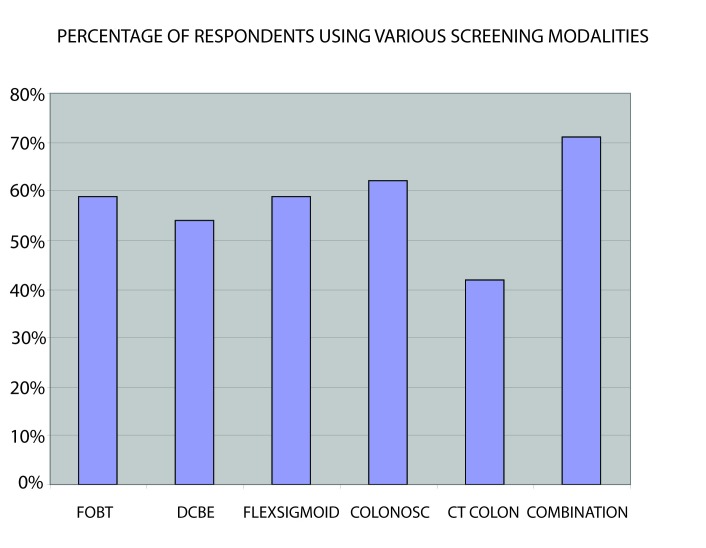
The screening methods. FOBT, Faecal occult blood test; DCBE, Double contrast barium enema; FLEX SIGMOID, Flexible Sigmoidoscopy; COLONOSC, Colonoscopy; CT COLON, CT Colonography; COMBINATION, Combination of 2 screening methods (Faecal occult blood test with double contrast barium enema or Sigmoidoscopy).
